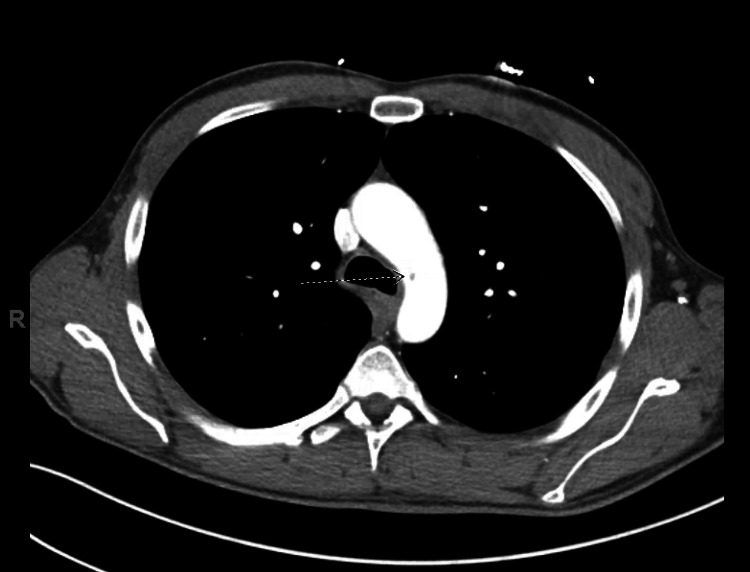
CT angiography of the aortic arch with the white arrow pointing at a small thrombus
